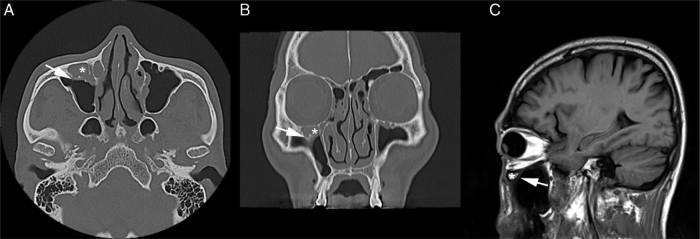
PNS CT revealed the presence of a soft tissue density in the right Haller's cell (*). The lesion abutted the infraorbital foramen (arrow) without definite evidence of communication. A, axial section; B, coronal section; C, sagittal section.
